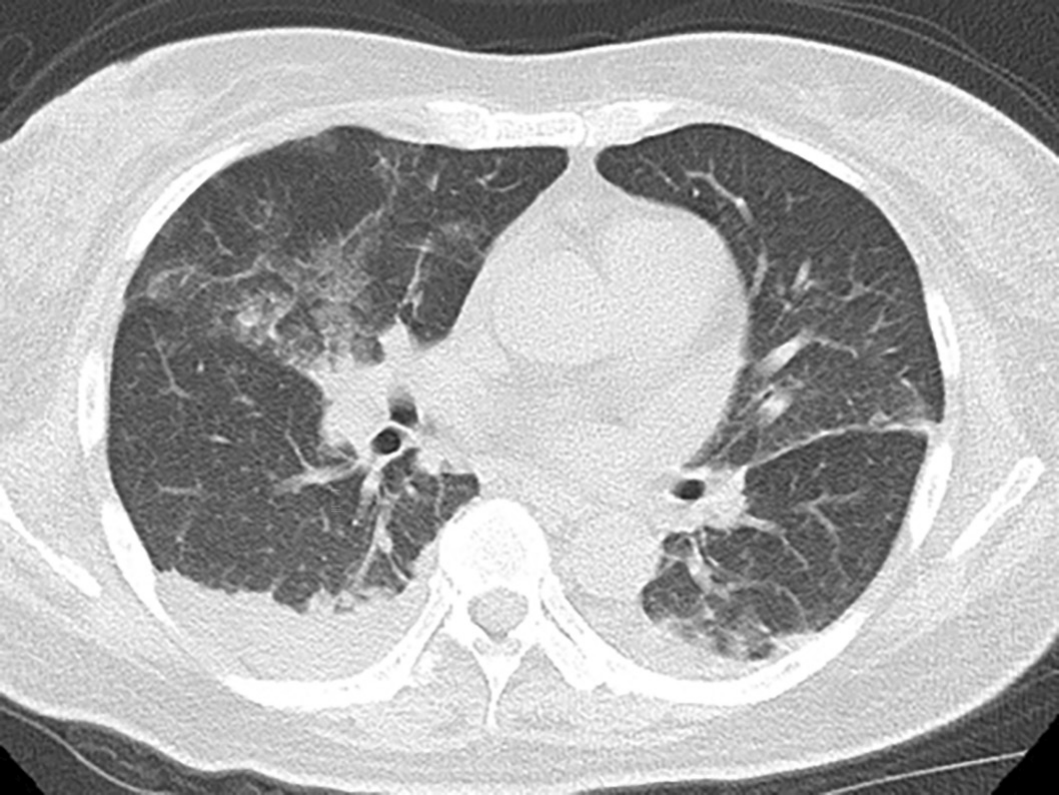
CT imaging of lungs. Ground‐glass opacities are observed in the bilateral lungs; however, no nodules suggestive of varicella pneumonia are found.
